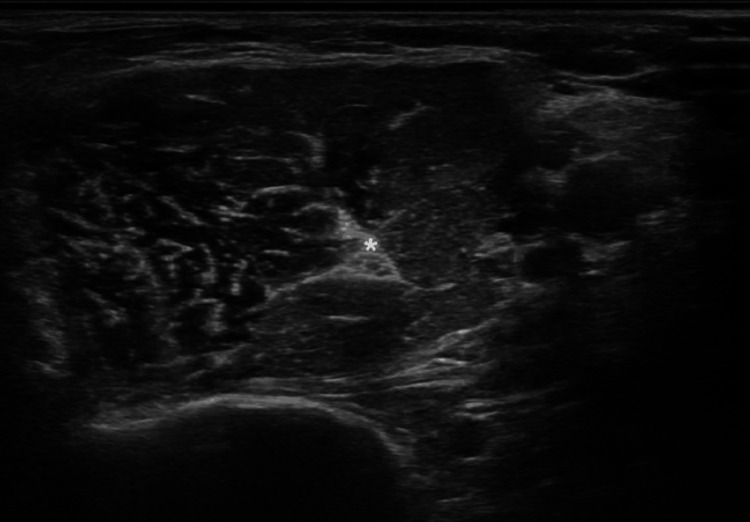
Transverse ultrasound section showing the musculocutaneous nerve (*) in the thickness of the coracobrachialis muscle.
